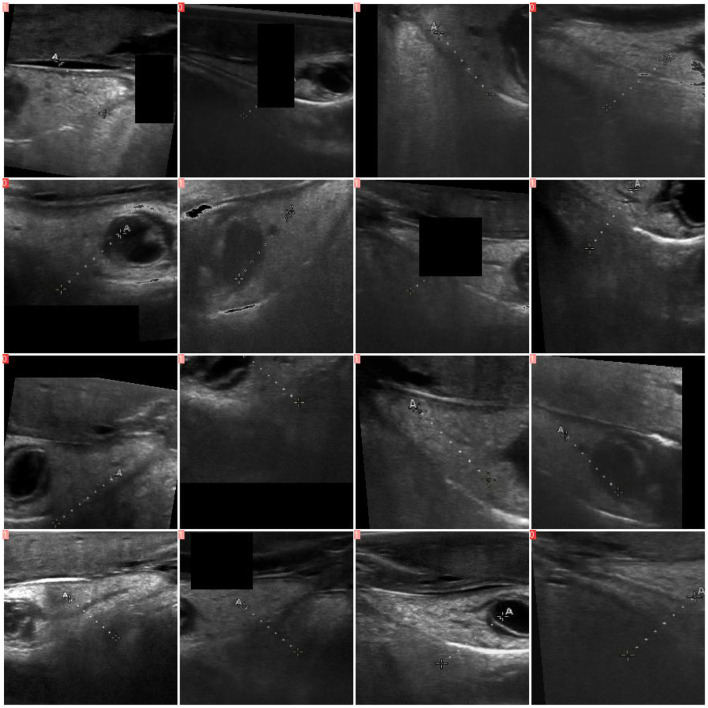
Examples of augmented red abalone (*Haliotis rufescens*) ultrasound images used during training. Augmentations applied according to YOLO defaults include random rectangle erasure (black regions), horizontal flipping, rotation up to 15 degrees, translation up to 10% of the image size, and brightness/contrast jittering. These transformations were applied uniformly across all model architectures to expand effective training diversity and reduce overfitting (Section 2.3.3).
